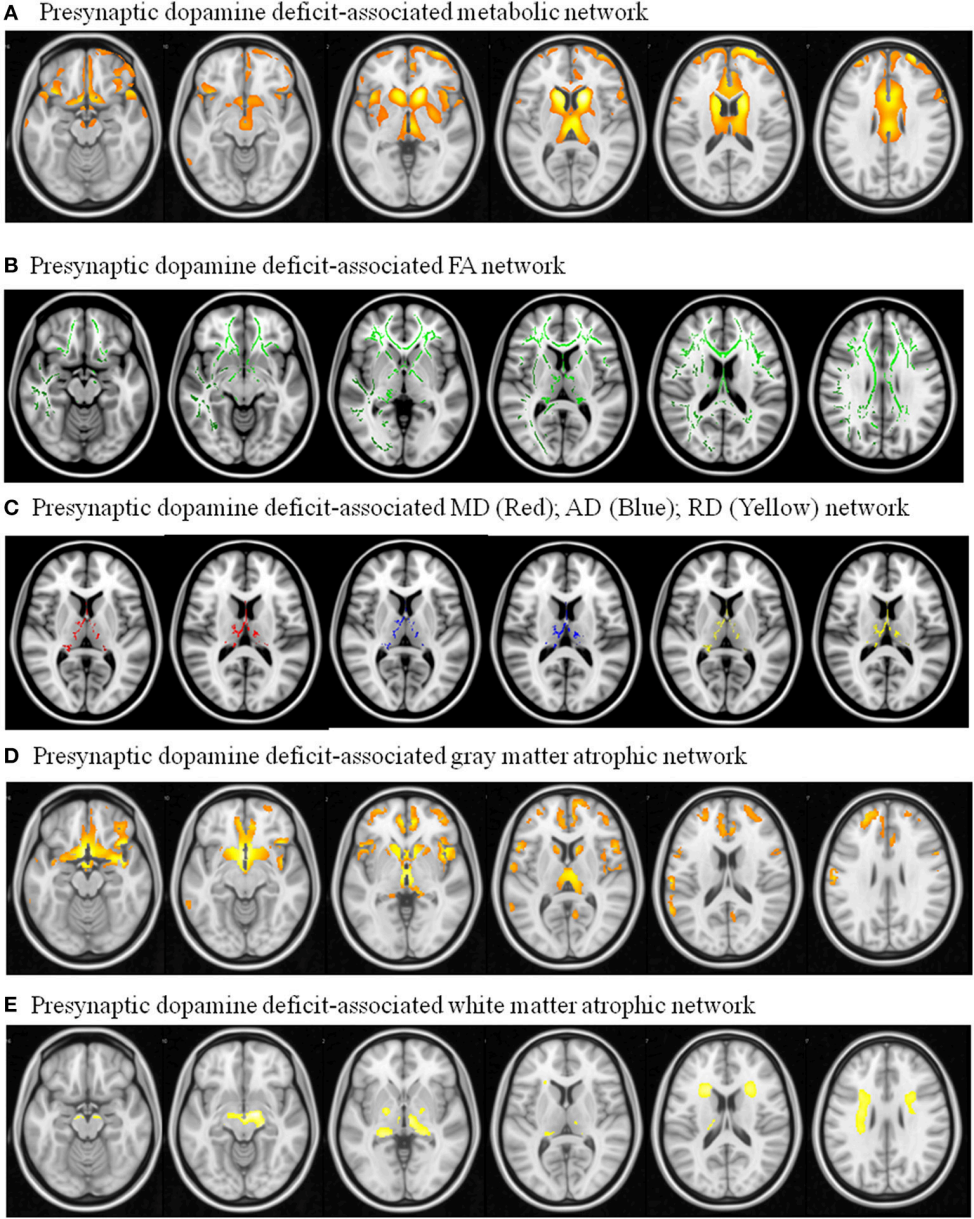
Pre-synaptic dopaminergic deficit-associated network with dependent variable using (A) ^18^F-fluro-2-deoxyglucose positron emission tomography ; (B) Fractional anisotropy (FA); (C) mean diffusivity (MD), axial diffusivity (AD), radial diffusivity (RD); (D) 3D T1-weighted segmented gray matter image; (E) 3D T1-weighted segmented WM image. Image results overlay on the T1 template.
